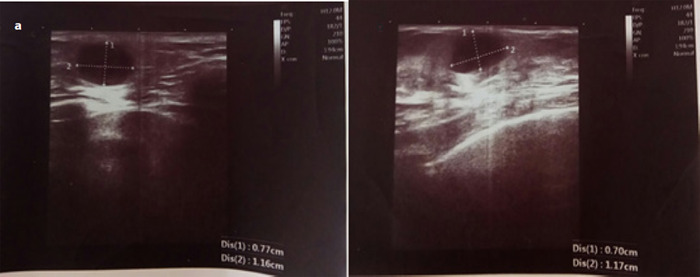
(a) Ultrasonography of the right breast, (b) Left Breast.
